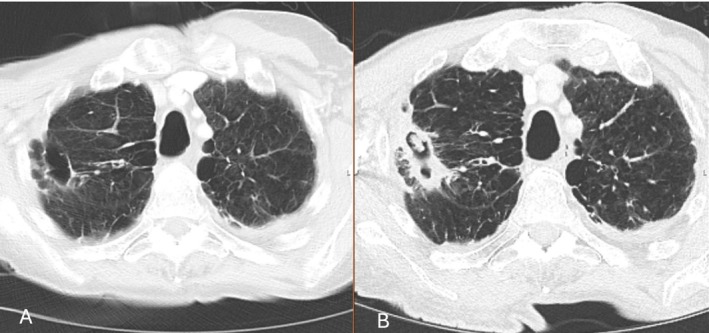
Follow‐up chest imaging of a 75‐year‐old man with COPD. (A) CT scan at end of antifungal treatment. (B) One year after stopping antifungal therapy, the scan shows reappearance of a fungal ball within the cavity, thickening of the cavity wall, and adjacent pulmonary consolidation.
